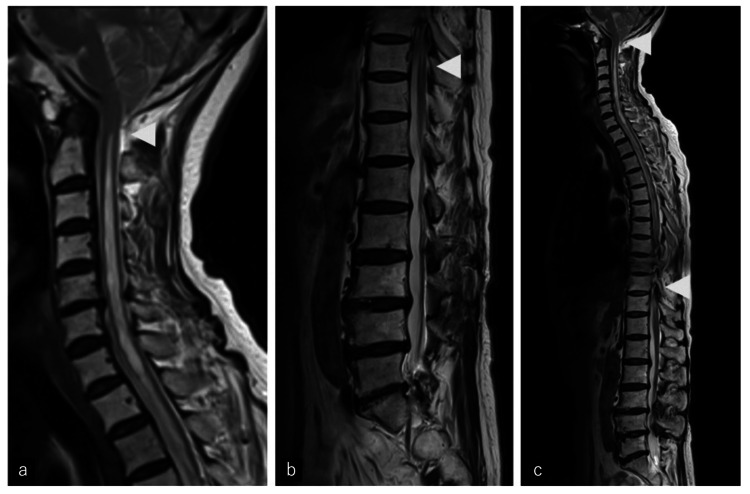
Spinal magnetic resonance imaging at the current presentation (a) Sagittal T2-weighted image of the cervical spine, (b) Sagittal T2-weighted image of the lumbar spine, (c)Sagittal T2-weighted image of the whole spine. The arrowhead shows an intramedullary T2-hyperintense lesion representing a syrinx, extending from the foramen magnum to T10 and showing marked longitudinal extension compared with the MRI obtained 21 years before.
